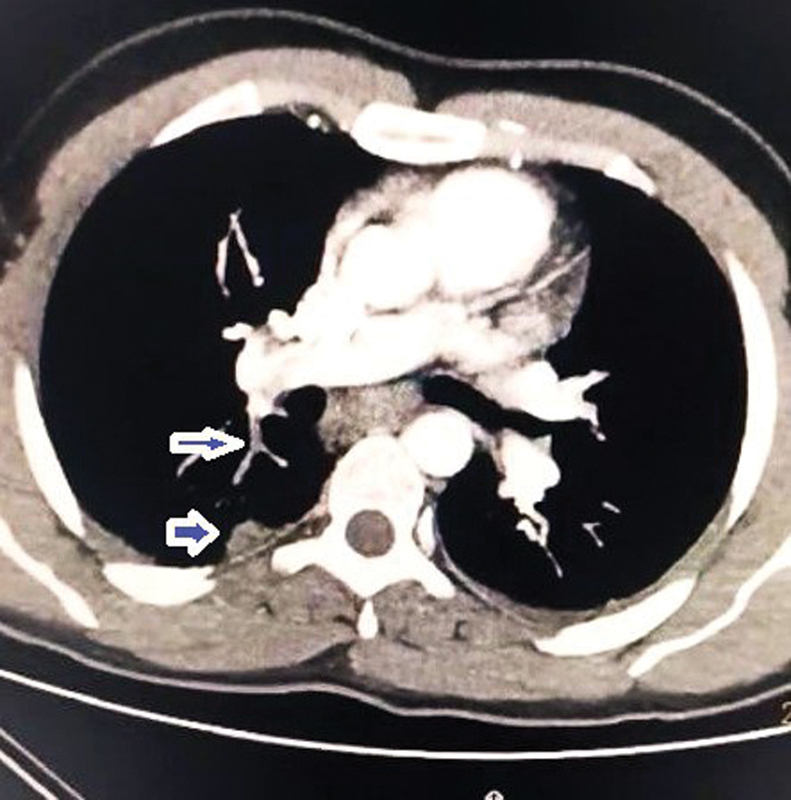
Thoracic computed tomography (CT) angiography revealed thrombus in the branches of the right pulmonary artery and pulmonary infarction. Arrows mark thrombosis in branches of the right pulmonary artery and a pulmonary infarction.
